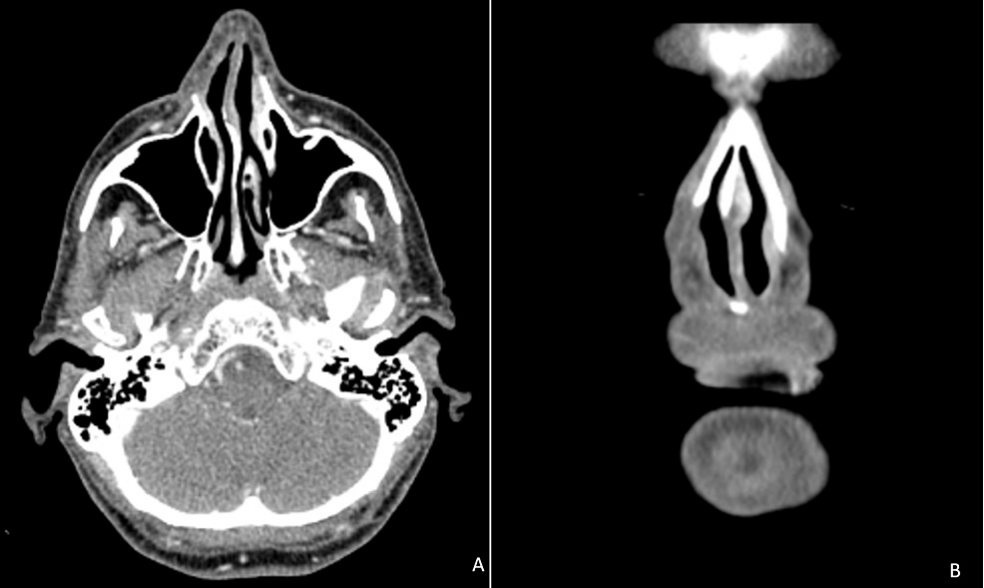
CT scans showing no signs of local recurrence A: axial view; B: coronal view CT: computed tomography
